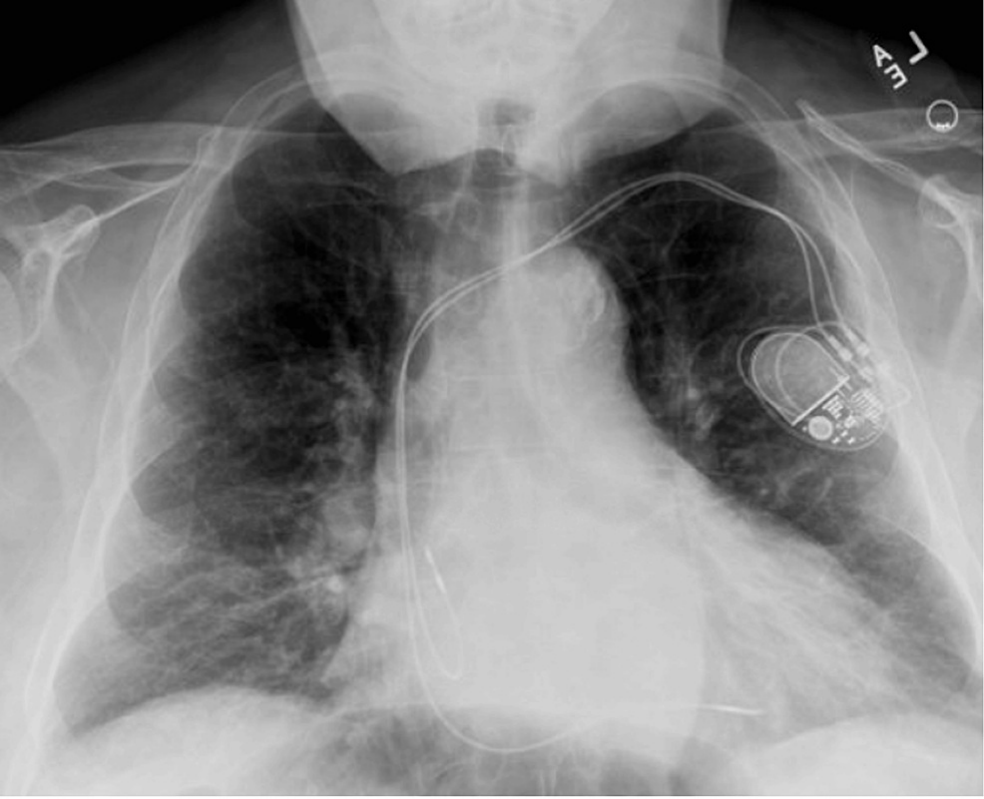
Post-procedure posteroanterior chest X-ray following right ventricular lead reimplantation (X-ray obtained on 12/5/2023)
